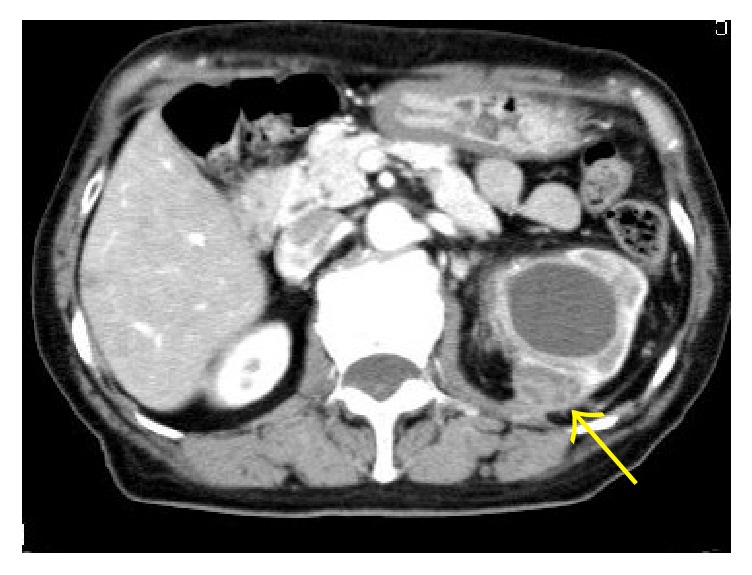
CT image: left renal mass with contrasted surface (arrow).
